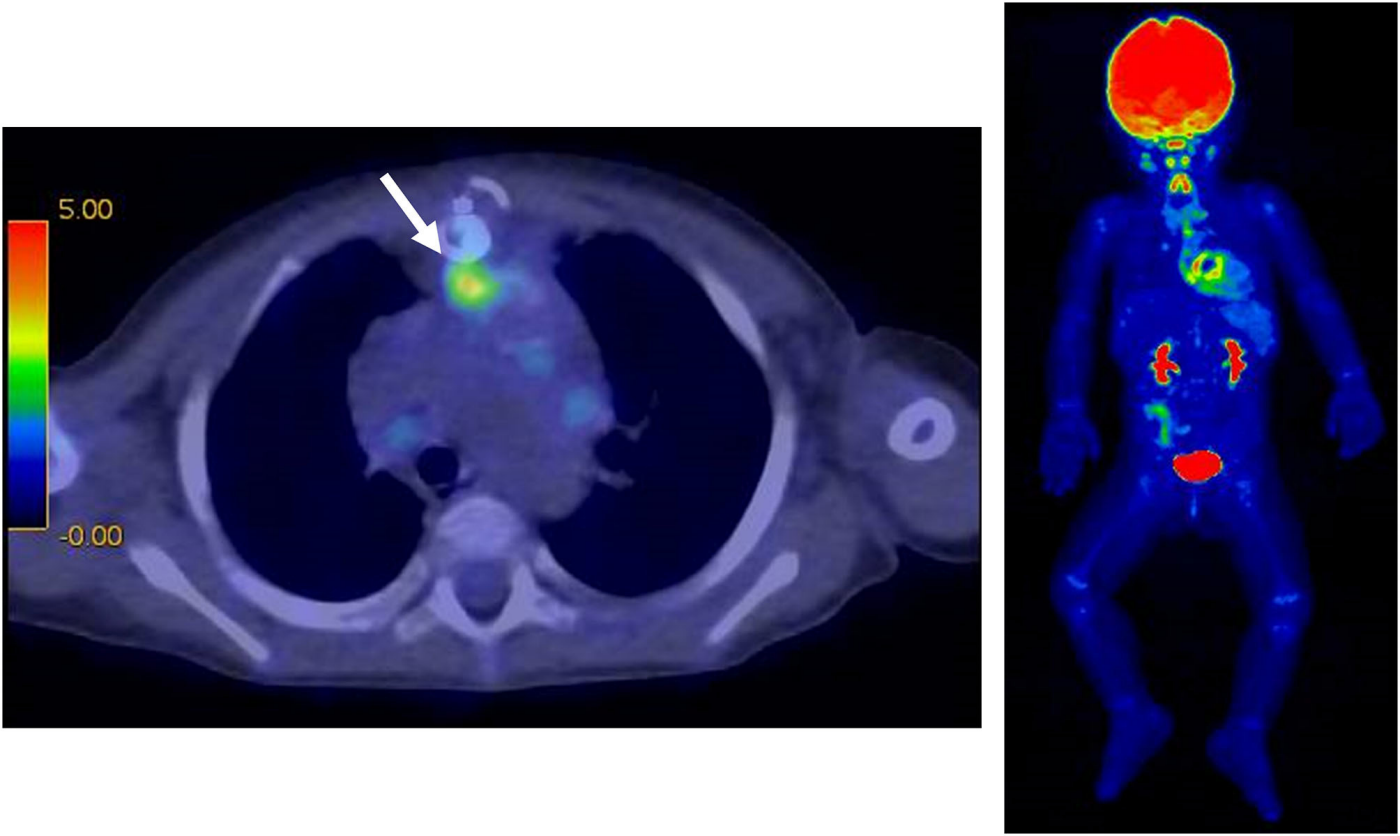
^18^F-FDG PET-CT at 115 days after surgery. There was a mild accumulation (SUV max 3.4) on the RV-PA conduit in the anterior mediastinum (white arrow).
